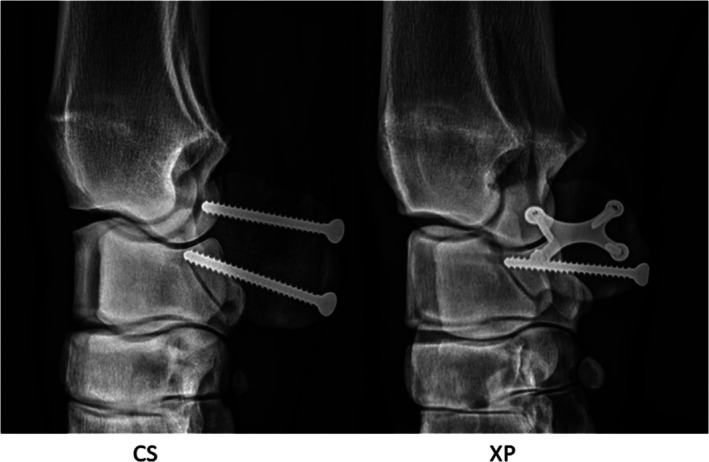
Postoperative radiographs of the accessory carpal bone (ACB) following fracture fixation. Left: Two 4.5 mm cortical screws in lag fashion (CS). Right: Medium X‐plate and 4.5 mm cortical screw in lag fashion (XP).
